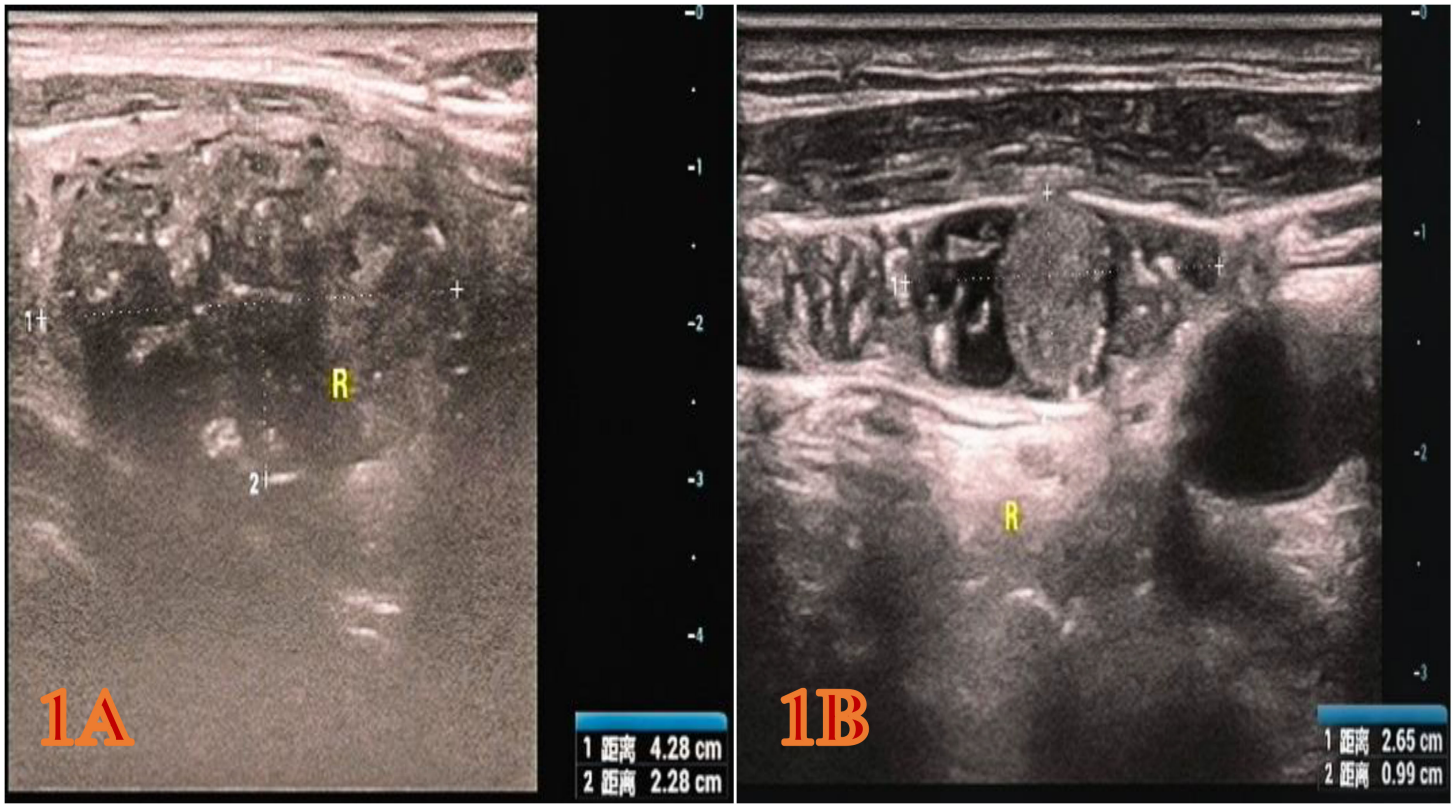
The color Doppler ultrasound image of the patient during the physical examination. (A) Right thyroid gland (hypoechoic nodule, 4.28x2.28x2.54 cm, heterogeneous hypoechoic, TI-RADS category 5). (B) Right cervical lymph nodes (the larger one approximately 2.65 x 0.99 cm, considered enlarged lymph nodes with structural abnormalities).
